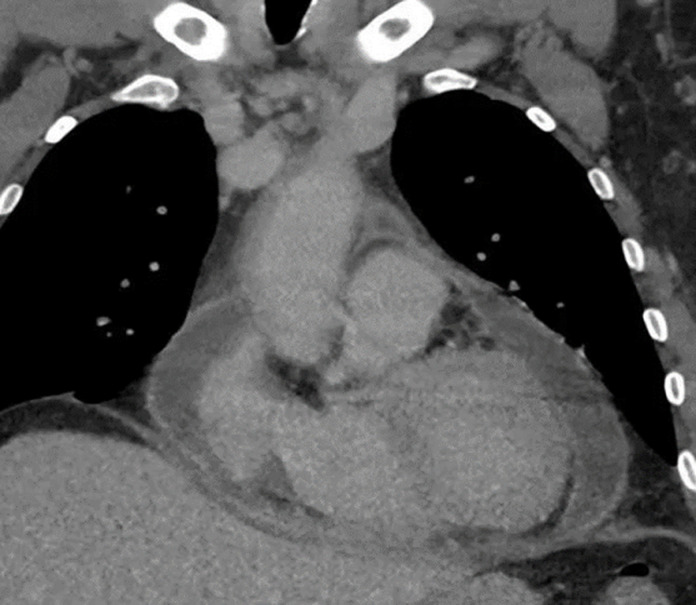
computed tomography scan of the chest showing the fluid collection within the pericardial sleeves
